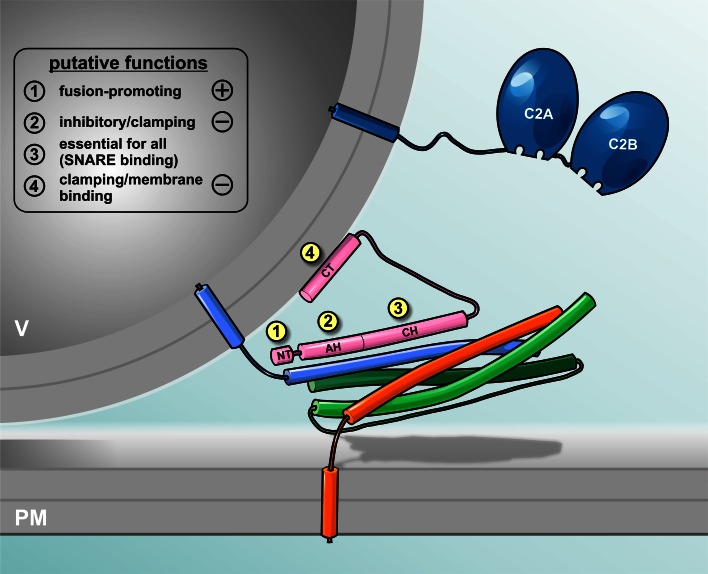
Hypothetical view on complexin and its interaction with the membrane-bridging SNARE complex. Vesicular SNARE (sybII, *blue*) and target SNARE (syx, orange and SNAP-25, *green*) partially assemble into trans-SNARE complex forming a high affinity binding site for complexin (*pink*). The N-terminus of Complexin (NT, amino acid 1–26) enhances fusion kinetics and fusogenicity [28, 45, 47, 48, 52, 63, 77, 78] while the accessory α-helix (AH, amino acid 27–47) [29, 41, 48, 49, 65–68, 71] and the C-terminus (CT, amino acid 73–134) [19, 34, 44, 50, 52, 63, 72] clamp premature release. The central helix (CH) of complexin binds with the SNARE complex [17, 20, 21, 27] which is prerequisite for all complexin actions [89]. The major Ca^2+^ sensor sytI (*blue*) interacts with SNAREs and membranes upon Ca^2+^-binding to its C2 domains, but is displayed separately for clarity of presentation
